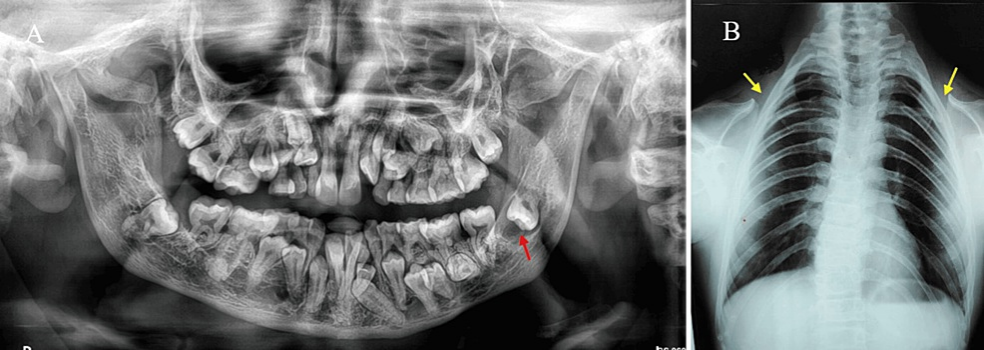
(A) Orthopantomography revealing multiple impacted permanent teeth and a dentigerous cyst around 38 in the ascending ramus of the mandible (red arrow). (B) Chest X-ray revealing the absence of a clavicle (yellow arrow)
